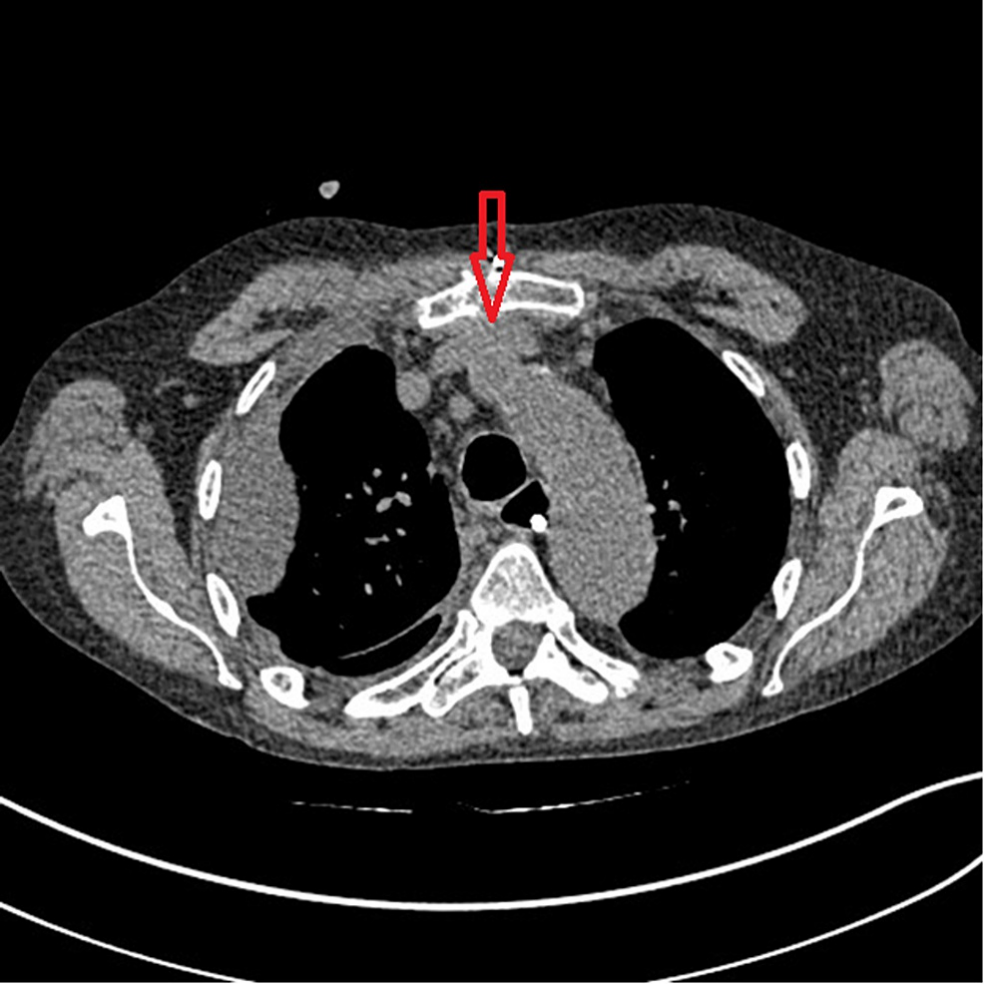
Non-contrast chest CT scan performed 10 days prior to venospasm demonstrating a normal-caliber brachiocephalic vein with no evidence of focal narrowing. CT: computed tomography
